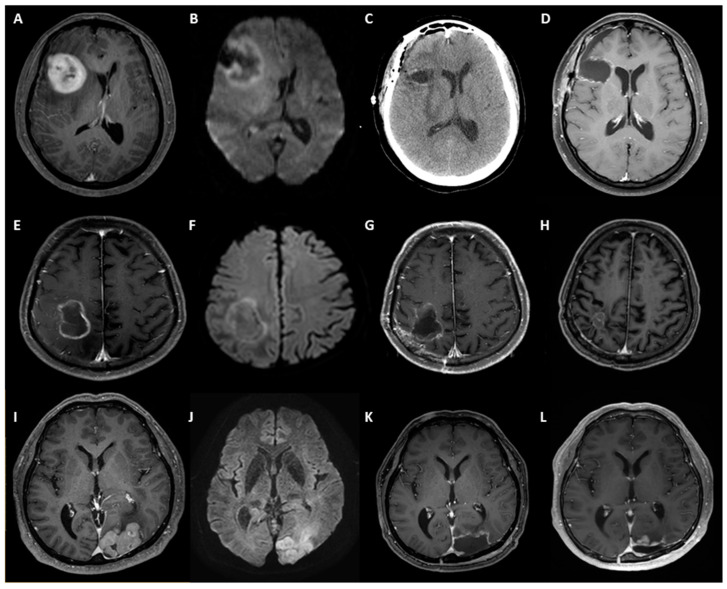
Different appearances of PCNSL in resected cases. One case with midline shifting presented with acute mental deterioration (A–D). (A) Preoperative T1 post-contrast image with clinical suspicion of PCNSL with significant midline shifting and neurological deterioration. (B) Diffusion-weighted image (DWI) of the same patient. (C) The immediate postoperative CT scan shows improvement in midline shifting. (D) T1 post-contrast image at 3 months after resection. Another case underwent resection owing to diagnostic ambiguity (E–H). (E) Preoperative T1 post-contrast image with clinical suspicion of high-grade glioma rather than lymphoma. (F) The DWI of the same patient shows mild diffusion restriction. (G) Immediate postoperative MRI after GTR. The frozen biopsy revealed high-grade malignancy without a definite identification of cell type. (H) T1 post-contrast image at 6 years after resection shows stable disease with CR. A case demonstrating an aggressive course following resection (I–L). Differential diagnosis from other malignancies, including extra-axial tumors, was needed based on preoperative T1 post-contrast image (I) and DWI (J). (K) GTR on the postoperative T1 post-contrast image. (L) A T1 post-contrast image was performed after the completion of the first induction CTx, which was 3 months after resection, indicating PD.
